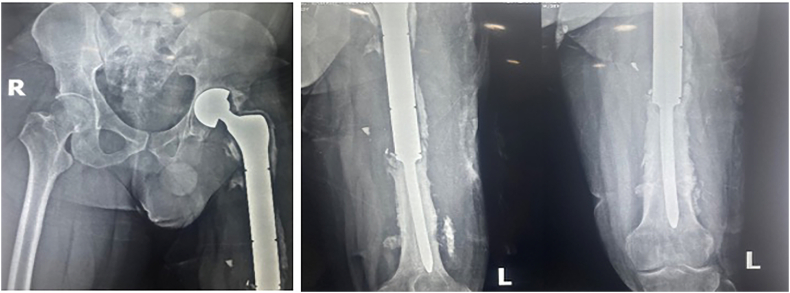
The pelvic X-ray and the left femur X-ray in the sixth year after installing the proximal femur megaprosthesis.
